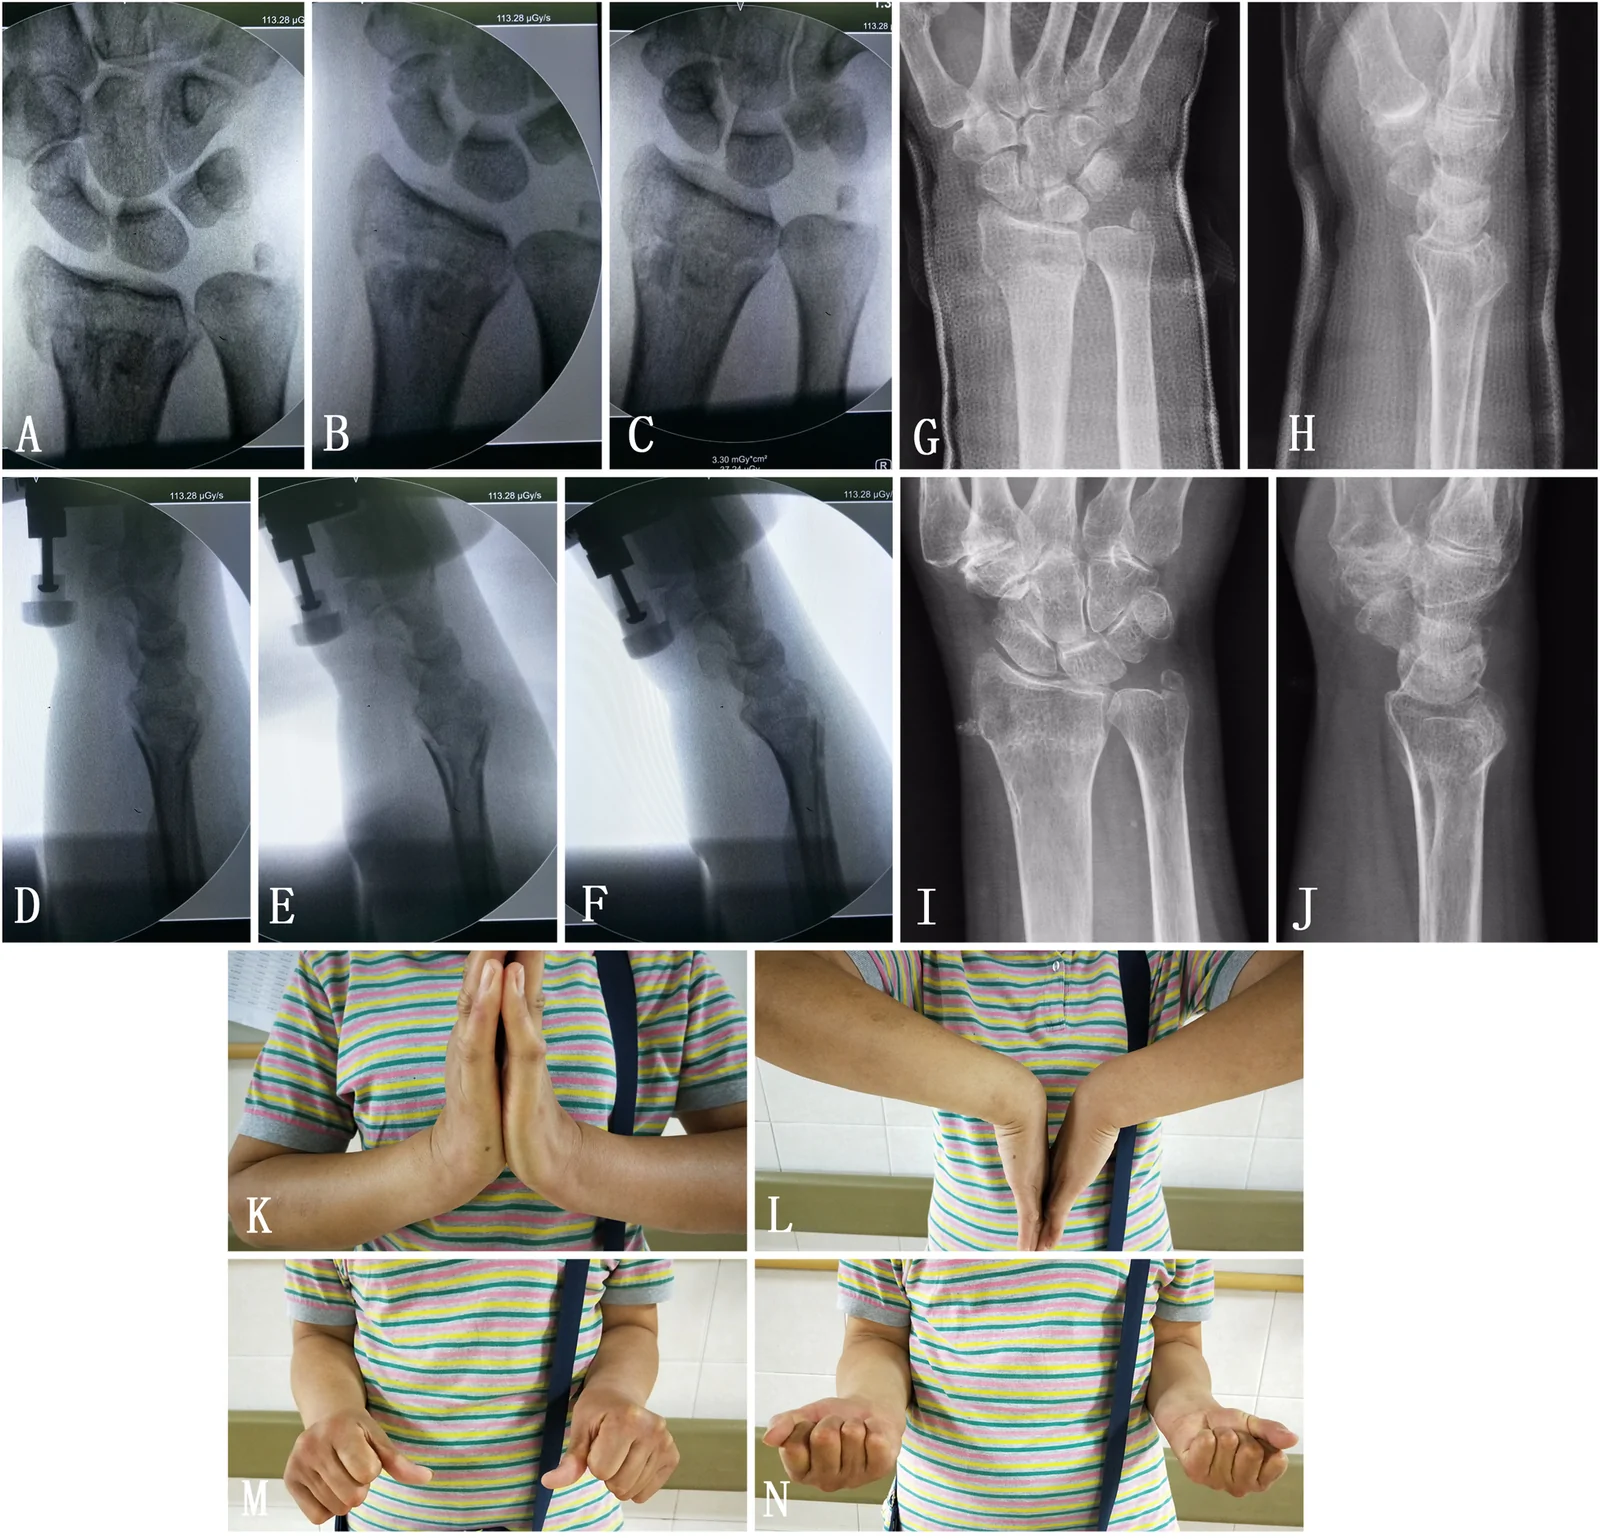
(A–C) the anteroposterior fluoroscopy image of fracture site when the distal fragment was gradually reduced by traction and ulnar deviation by operating the device. (D–F) The fluoroscopy image of fracture site when the distal fragment was gradually reduced by flexion by operating the device. (G–H) The immediate x-ray of fracture after reduction and cast immobilization. (I,J) The x-ray of fracture at 6-month follow up. (K–N) The ROM of injured wrist at 6-month follow-up.
